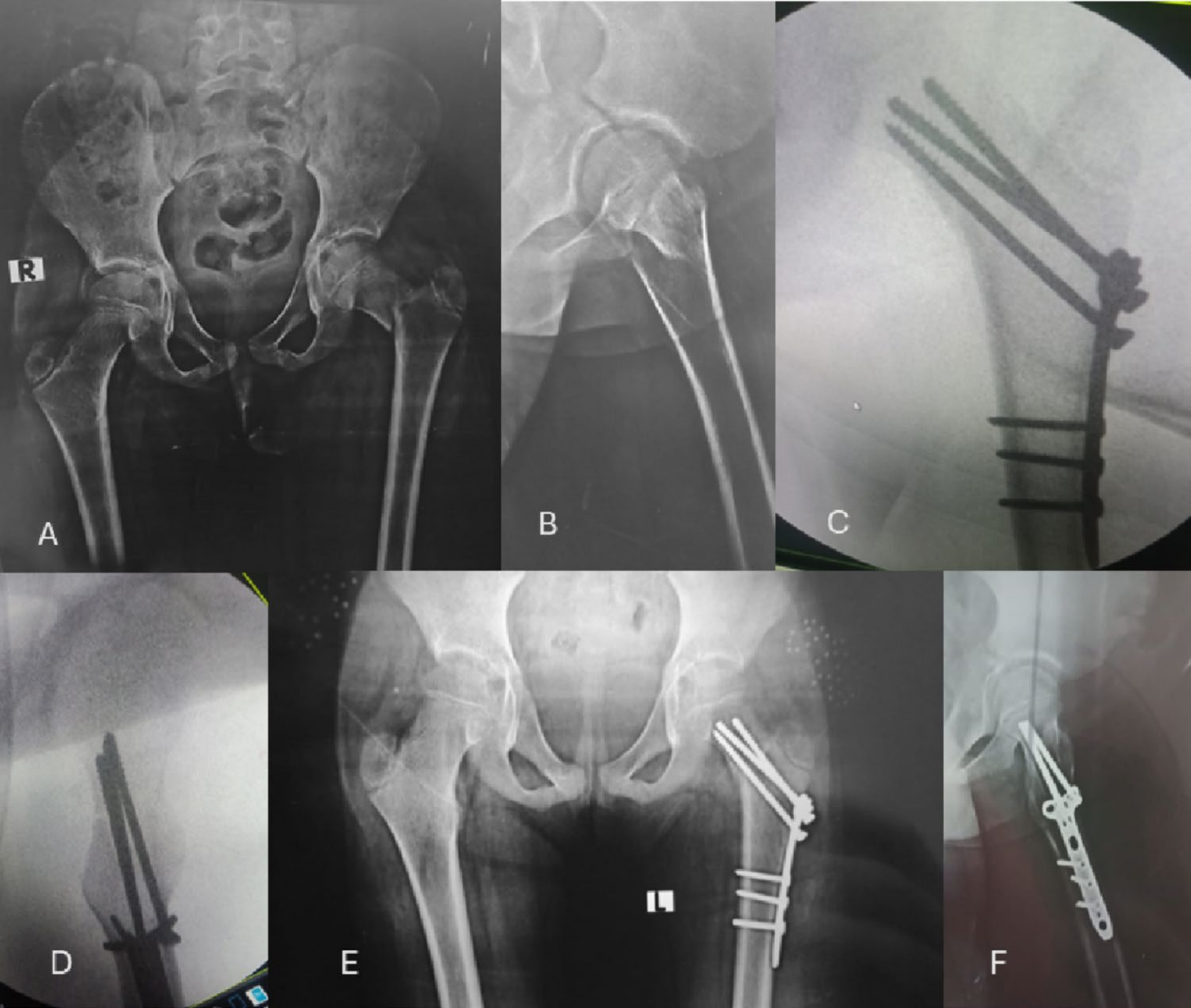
A and B show an X-ray anteroposterior view of both hip joints and a lateral view of the left hip joint showing a Delbet type III proximal femoral fracture. C and D show Intraoperative Fluoroscopic images showing intraoperative reduction and fixation using a contoured 3.5 mm T-plate. E and F Follow-up X-ray 6 months postoperatively showing the maintained hip radiographic parameters with healing of the fracture
